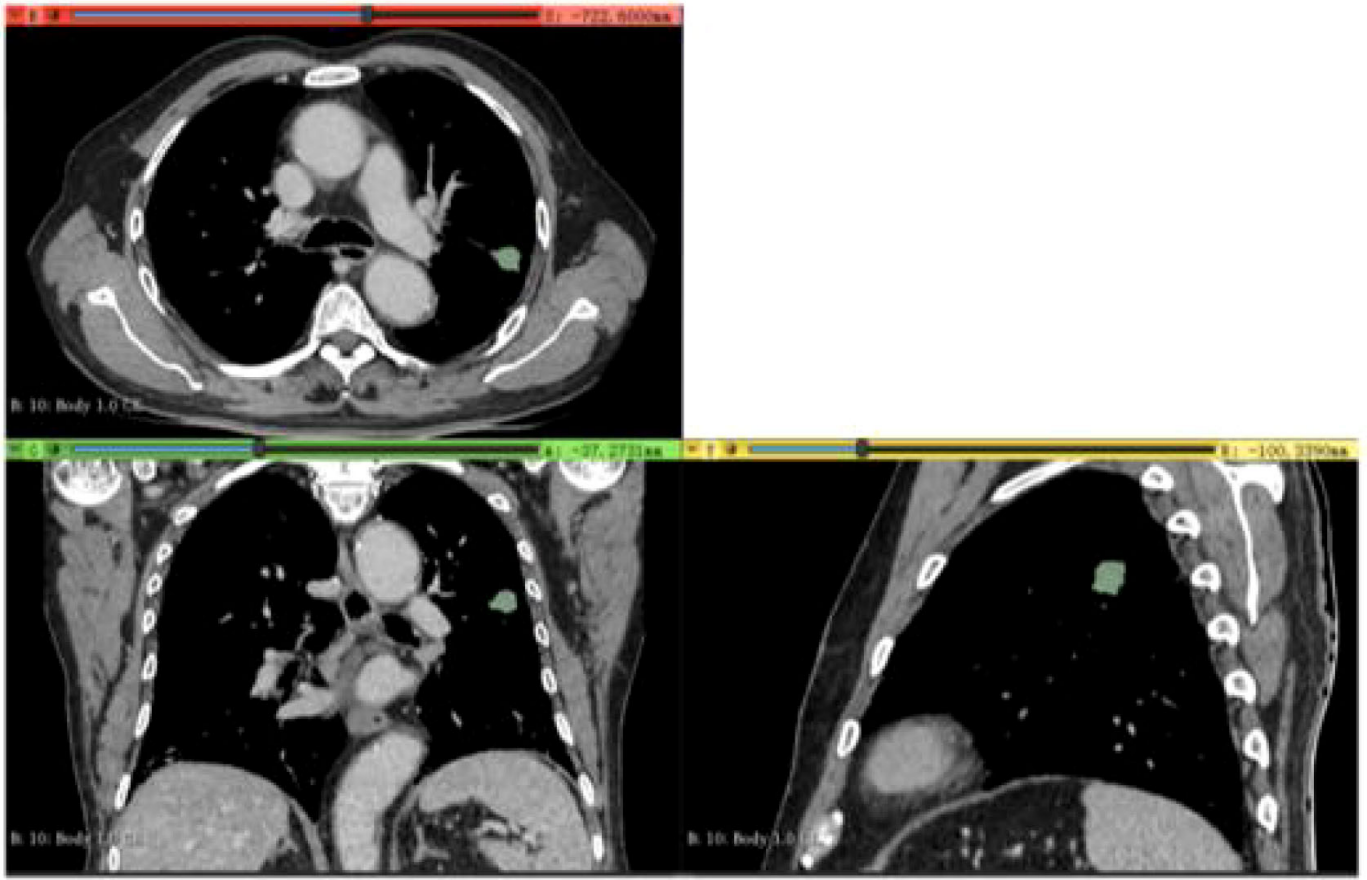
Delineation of Region of Interest (ROI) in a 65-Year-Old Male Patient (Validation Cohort). Delineation of region of interest (ROI) in a 65-year-old male patient (validation cohort) using portal venous phase CT scan exported from 3D Slicer software. Axial, coronal, and sagittal views with three-dimensional ROI reconstruction (green area) are shown.
